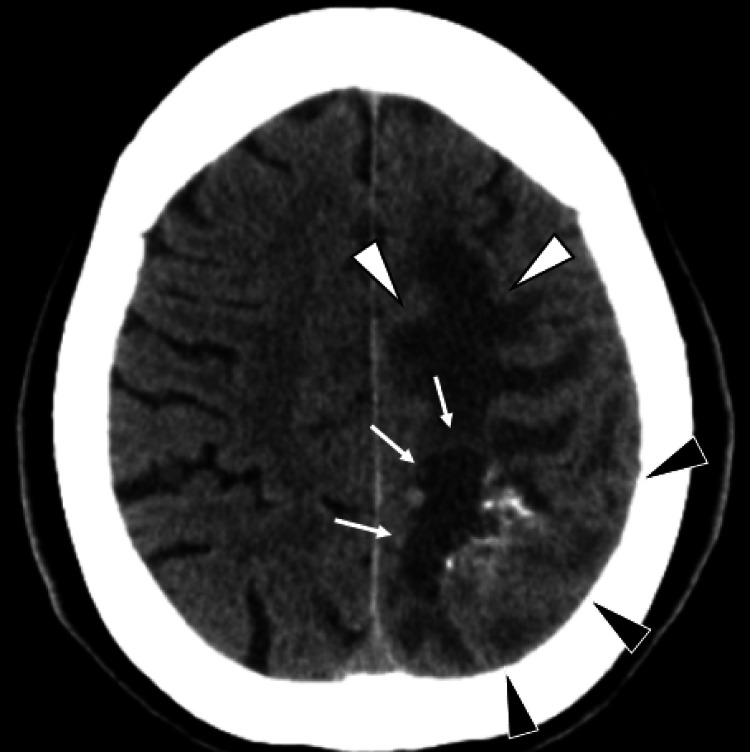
Brain CT findings. Axial non-contrast-enhanced brain CT image showing a 30 × 35 mm tumor in the left parietal lobe composed of an isodense solid component (black arrowheads) and a hypodense cystic component (white arrows). Peritumoral edema is visible (white arrowheads), but no midline shift is present.
